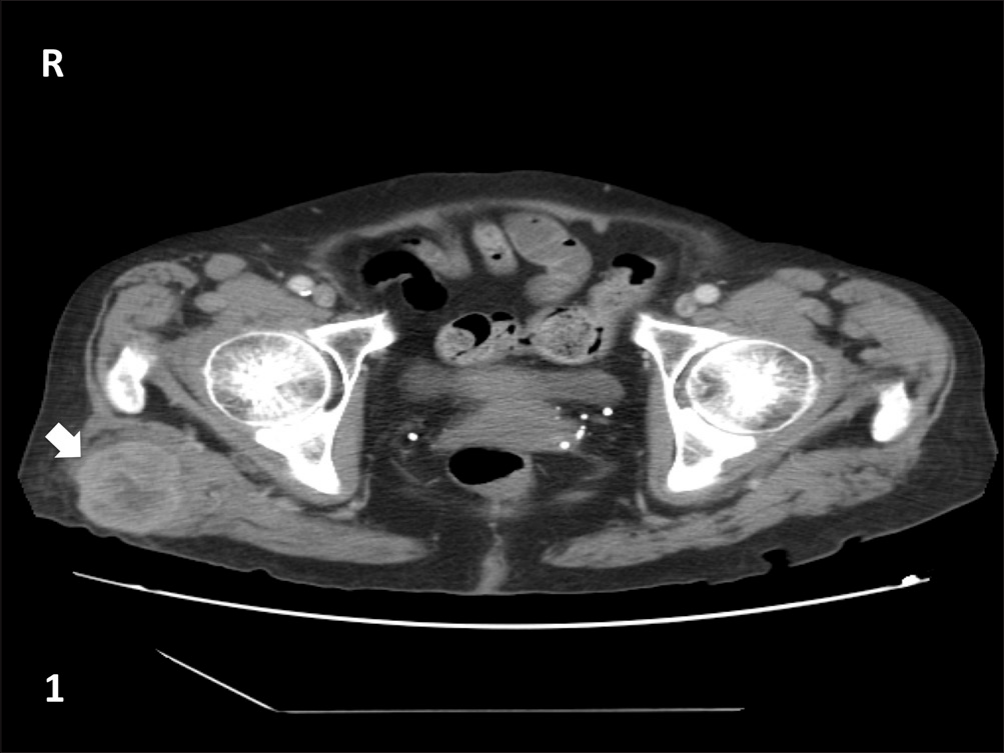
CT imaging of the pelvis with contrast. A contrast enhancing mass was identified in the right intra-gluteal muscle and measured 4.4 × 3.8 cm (arrow)
